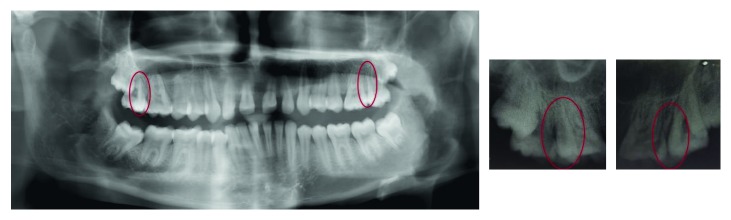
Panoramic and intraoral radiographs showing bilateral maxillary paramolars (encircled).
